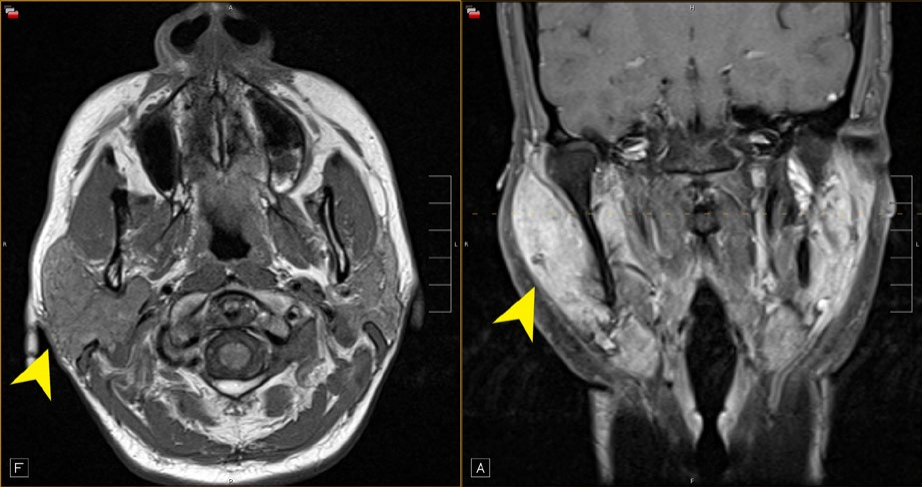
MRI revealed asymmetric enlargement of the right parotid gland involving both superficial and deep lobes
